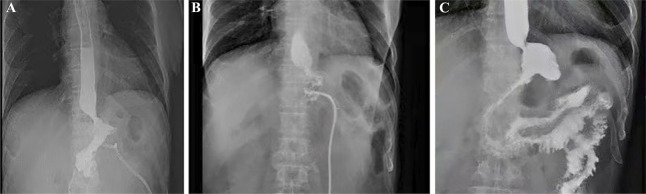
Results of upper gastrointestinal contrast examination. (A) On February 5, 2021, the patient underwent upper gastrointestinal radiography, and the contrast agent could be seen leaking from the fistula. (B) On February 18, 2021, the patient underwent upper gastrointestinal radiography, and the contrast agent could be seen entering the esophagus from the fistula. (C) On March 10, 2021, the patient underwent upper gastrointestinal radiography again, and no leakage of contrast agent was observed.
